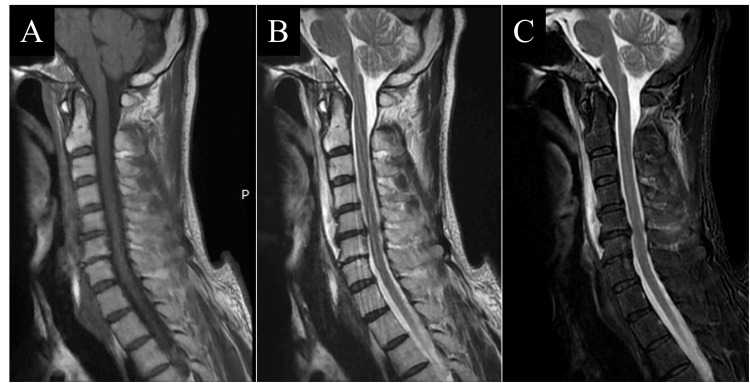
Cervical spine MRI on the day following the injury (day X+1). A: Sagittal T1-weighted image. B: Sagittal T2-weighted image. C: Sagittal short tau inversion recovery (STIR) image. In A–C, signal changes within the bone were observed at the occipital base, C4 vertebral body, and C4 spinous process. Similarly, hematoma formation was noted in the anterior longitudinal ligament in front of the cervical spine. However, no signal changes were observed within the intervertebral discs or the spinal cord.
